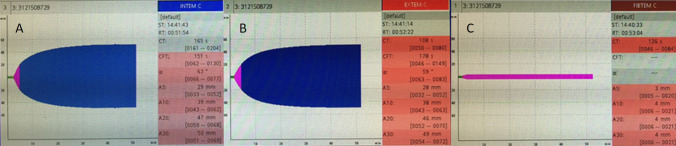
a–c Rotational Thromboelastometric analysis in citrated blood samples (ROTEM®, TEM International, Munich, Germany) showing the clot formation (mm) over time (s) at the beginning of surgery. The INTEM® (phospholipids and ellagic acid to initiate intrinsic clotting; (a) and EXTEM® (tissue factor to initiate extrinsic clotting; (b) analysis with prolonged clot formation times (CFT, seconds) and reduced clotting amplitudes after a clotting time (CT, seconds) of 5, 10, 20, 30 min (A5-A30; mm) and clot formation angle (α, degrees) reflect the concomitant consumption of clotting factors for the intrinsic and extrinsic pathway of coagulation. The reduced amplitudes of the fibrinogen related clot formation at different time points (A5-A30) as well as the prolonged CT in the FIBTEM® Test (Inhibition of platelet activity using cytochalasin to reflect clot tracing dependent on fibrinogen; (c) show the reduced functional fibrinogen fraction in clot formation
